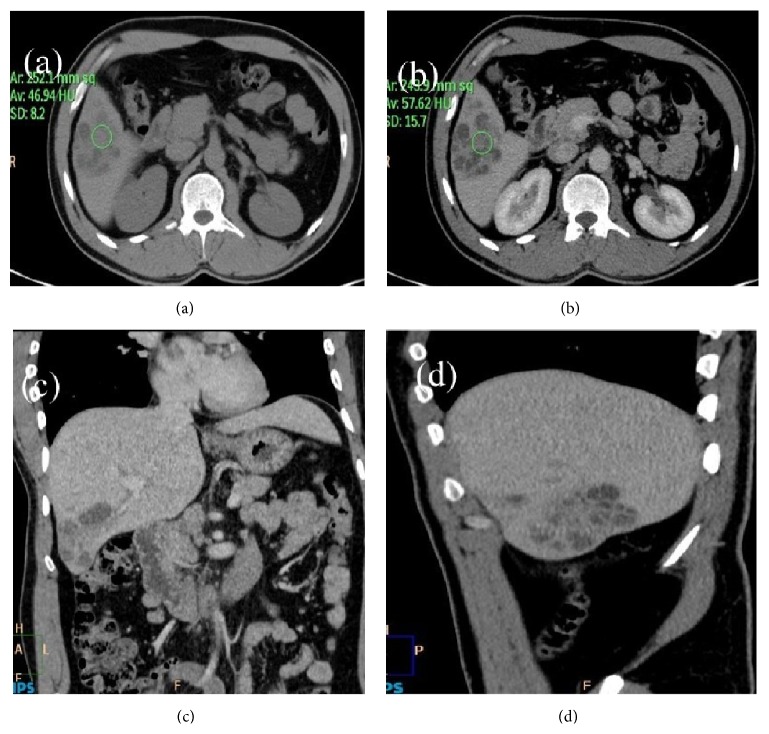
(a) Plain CT image of abdomen: axial view showing ill-defined, mixed density mass lesions in segment V of liver. Hounsfield unit at noncystic part is 47 in the center of the lesion. (b) Postcontrast CT image of abdomen: axial view showing ill-defined heterogeneously enhancing conglomerated mass lesions in segment V of liver. Hounsfield unit at noncystic part is 57 in the center of the lesion. (c) Postcontrast CT image of abdomen: coronal view showing ill-defined conglomerate mass lesions in segment V of liver. Remainder of abdomen and visualized part of lungs are devoid of any significant pathology. (d) Postcontrast CT image of abdomen: sagittal view showing ill-defined conglomerated lesions in segment V of liver. There is mild enlargement of the liver.
